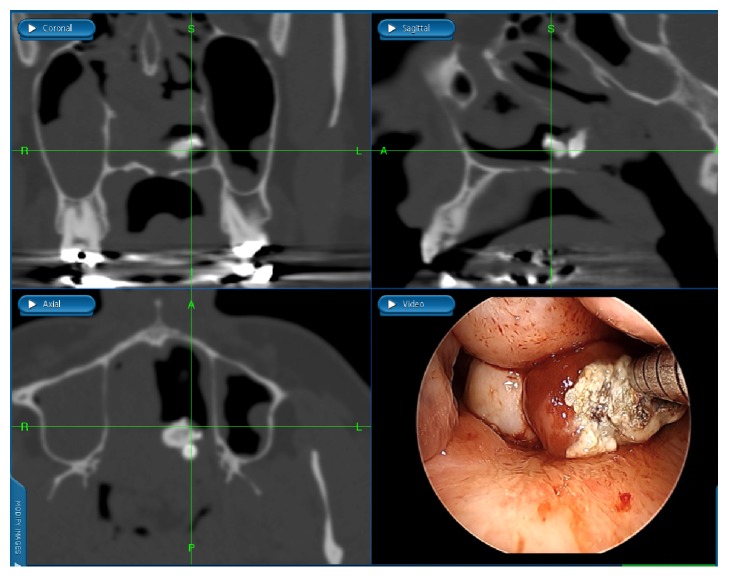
Use of intraoperative CT image guidance assistance during skull base approach. Computer-assisted navigation system (image guidance) highlighting intraoperative CT scan imaging in coronal, sagittal, and axial views. With the registered instrument tip contacting the anterior face of the lesion (endoscopic image at bottom right), the fine cut maxillofacial CT scan shows calcified material present within the skull base teratoma at this site of tumor contact (intersection point of green lines), representing intratumoral dentition.
